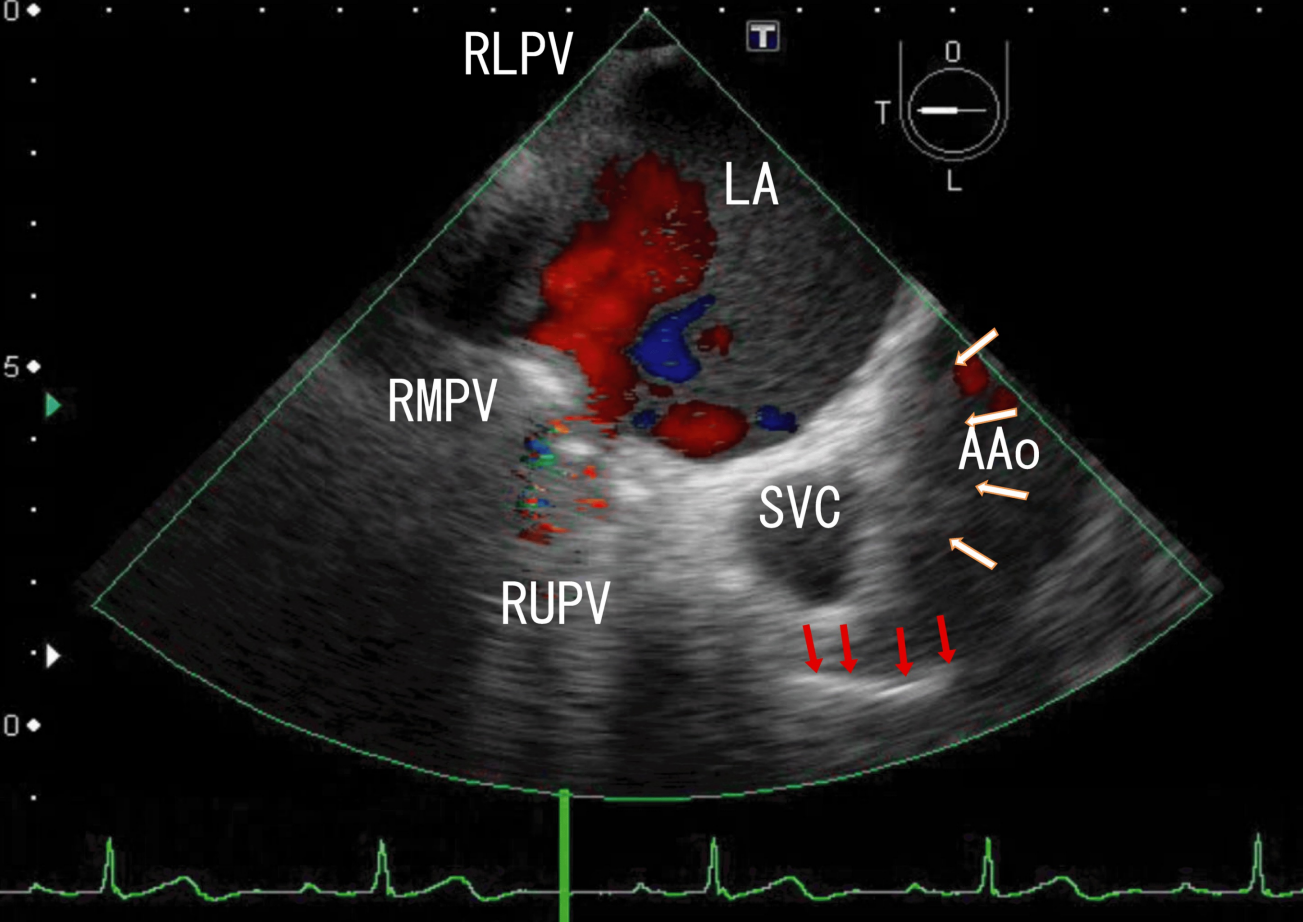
TEE images showing AAo thrombi and approaching several white parts TEE revealed small thrombi in the AAo (white arrows), with the attachment areas located on the upper side. Most thrombi in the AAo resolved, and the LA thrombi could have been small. The red blood flow from the RMPV increased slightly. The small red area represents blood flow from the RLPV. The LA thrombi from the RLPV became larger. Short, line-like white thrombi could be identified (red arrows). TEE: transesophageal echocardiography, AAo: ascending aorta, LA: left atrium, SVC: superior vena cava, RLPV: right lower pulmonary vein, RUPV: right upper pulmonary vein, RMPV: right middle pulmonary vein
